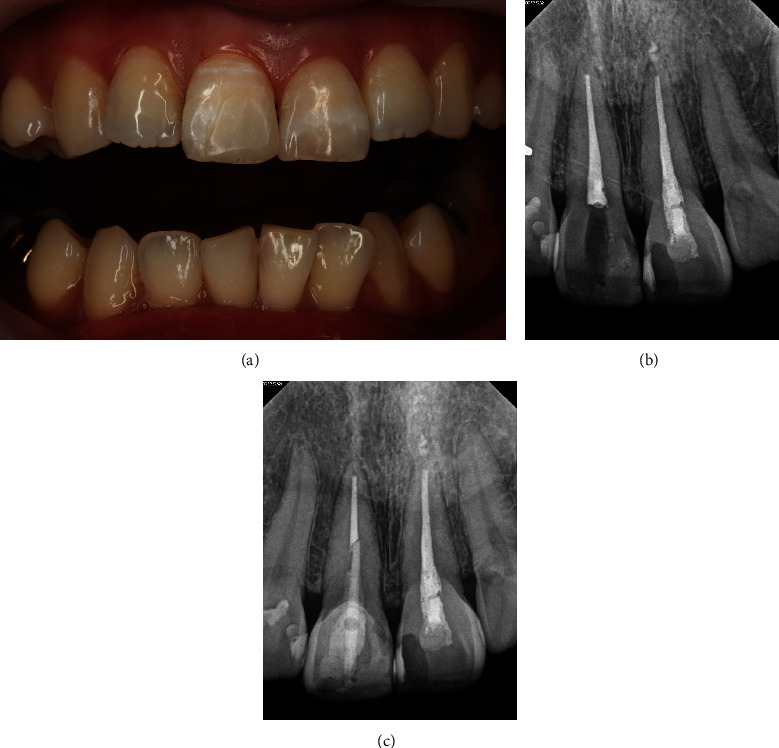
(a) Intra-oral photograph after post placement and resin build-up. (b) Periapical view after canal filling. (c) Periapical view after post placement, and resin build-up.
